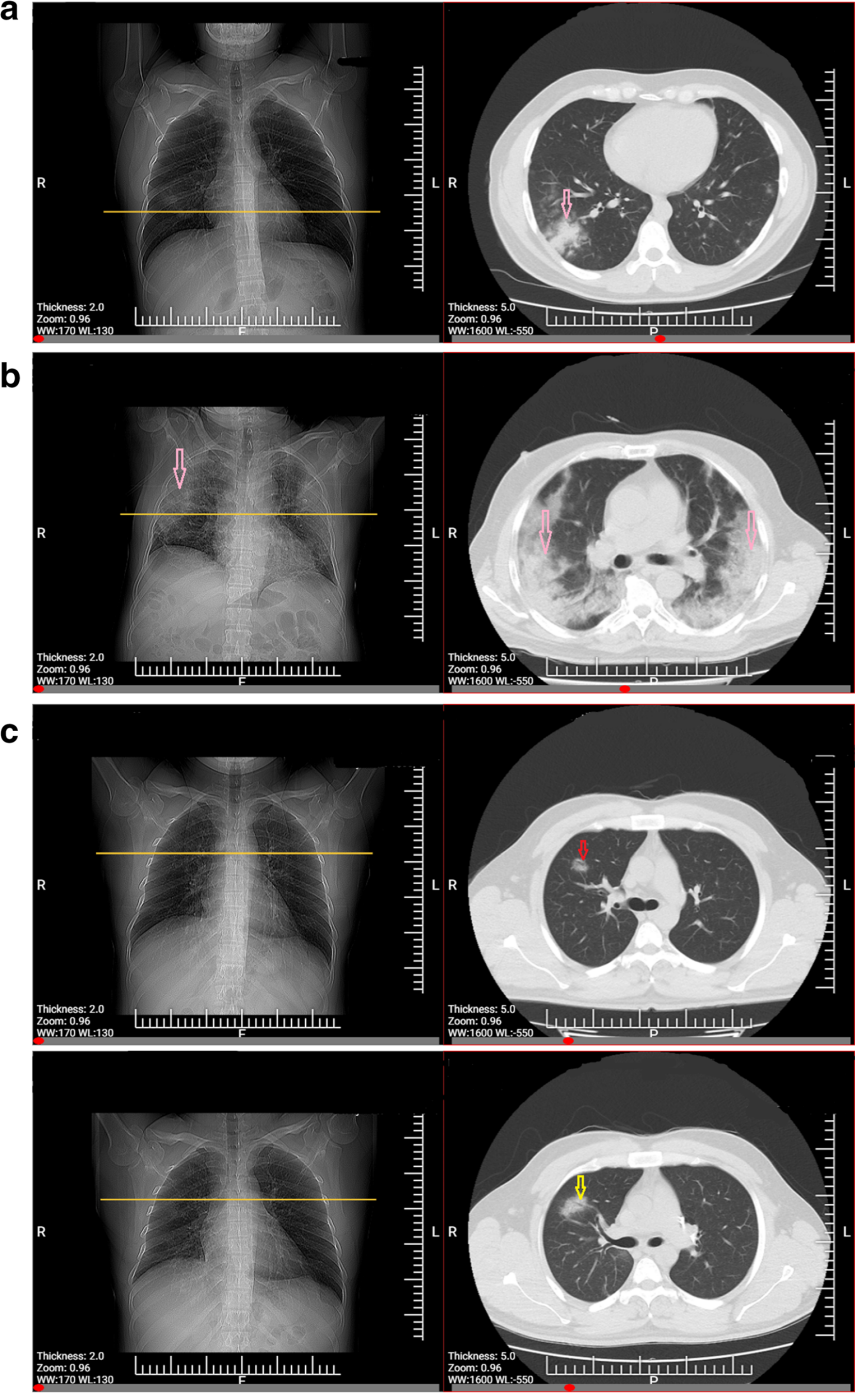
a: CT findings of confirmed COVID-19 pneumonia. Consolidation pattern, a young patient presented with fever (38.6 °C) and exposure history. Chest CT acquired on March 28, 2020 showed consolidation lesions (arrow) in the lobe of right lung. b: CT findings of severe type confirmed COVID-19 pneumonia. A old patient presented with fever and respiratory occurs and requires mechanical ventilation in ICU. Chest CT was got on the same day as positive RT-PCR with distributed ground-glass opacities with consolidation (arrows) in the lobes of right and left lung. c: CT findings of confirmed COVID-19 pneumonia. Ground-glass opacity (GGO) pattern, A young patient presenting without fever with lab-confirmed COVID-19. I: Chest CT acquired 2 days before the first positive RT-PCR test showed a rounded GGO in the right lung upper lobe (arrow). II: Follow-up chest CT-Scan after 3 days showed the size enhancement of the lesion (arrow)
